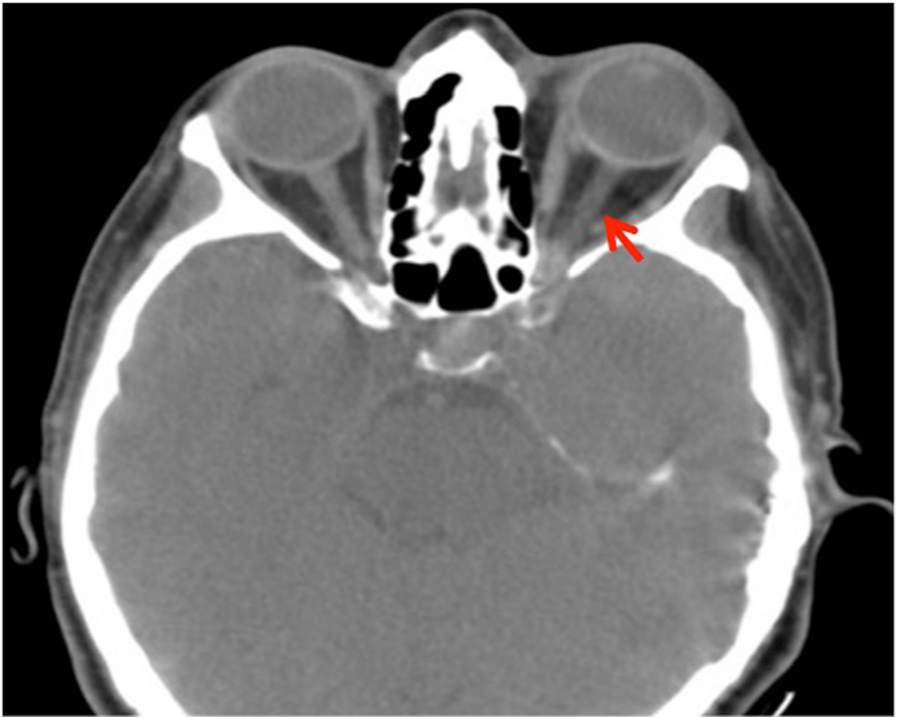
Computed tomography of the brain. Regular diameter of the superior ophthalmic vein on the *left* (arrow) and *regular* signal of the fatty periorbital tissue
